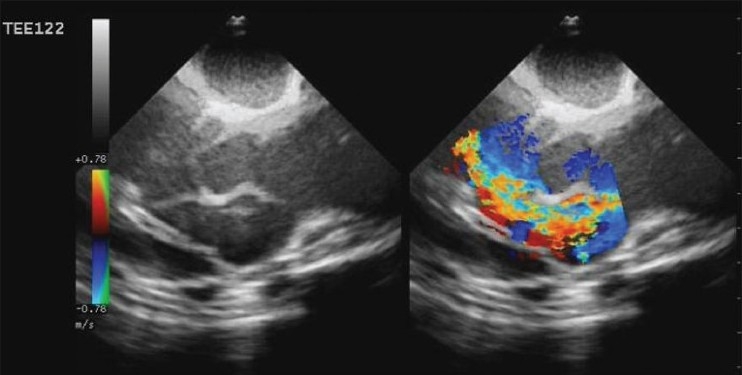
Two-dimensional and color flow imaging of the tunnel in transesophageal echocardiographic examination
